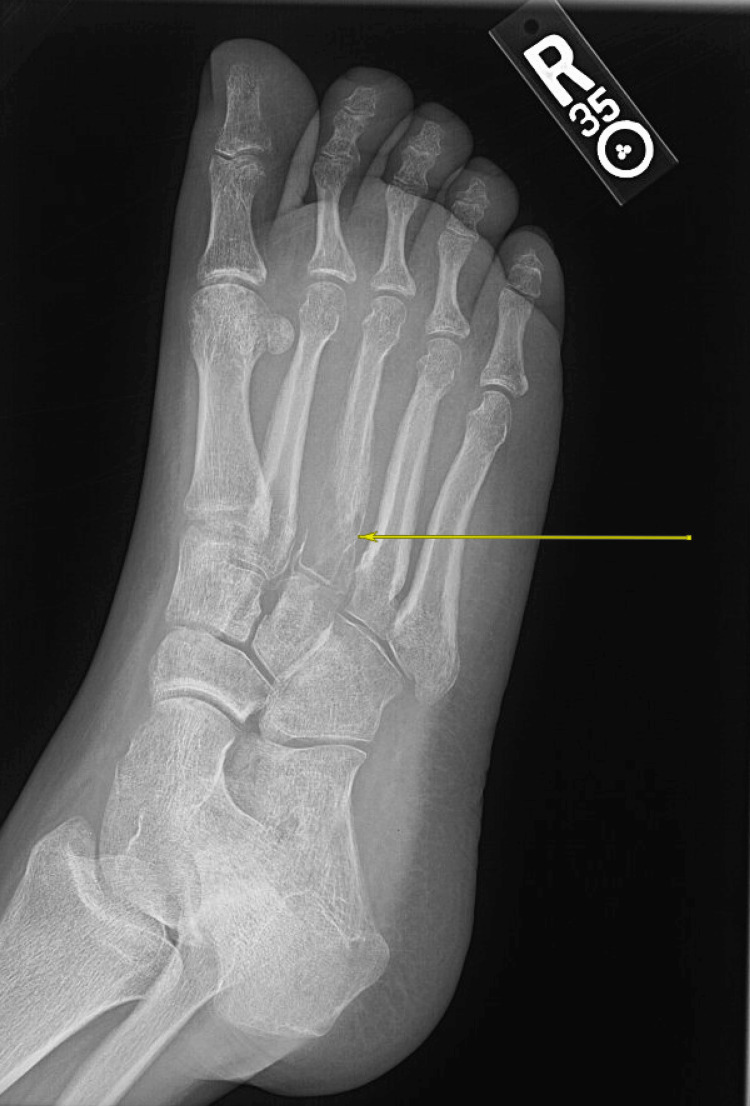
Foot X-ray demonstrated focal osteopenia with extensive resorptive changes of the proximal third metatarsal with periosteal thickening and overlying soft tissue swelling, most concerning for osteomyelitis. The arrow indicates the focal area of osteopenia of the proximal third metatarsal.
